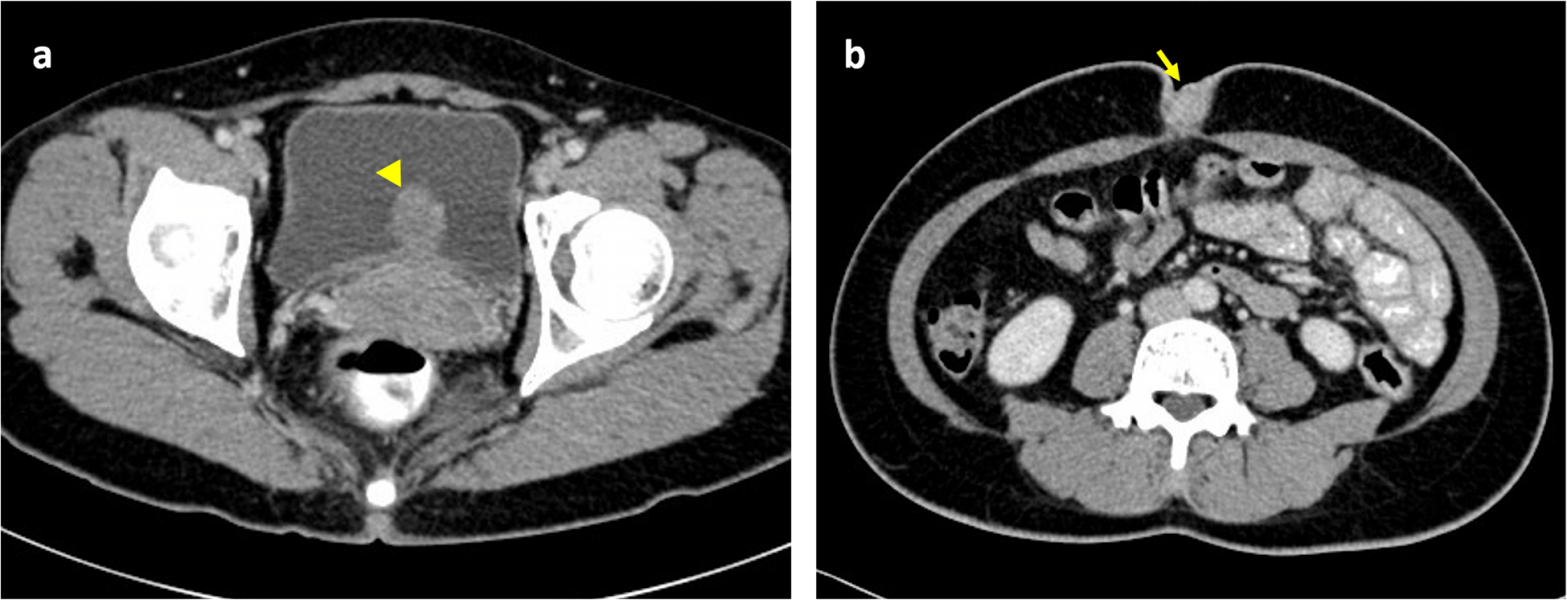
Computed tomography revealing a. A protruding mass on the posterior wall of the urinary bladder (arrowhead). b. A mass in the umbilicus (arrow)
